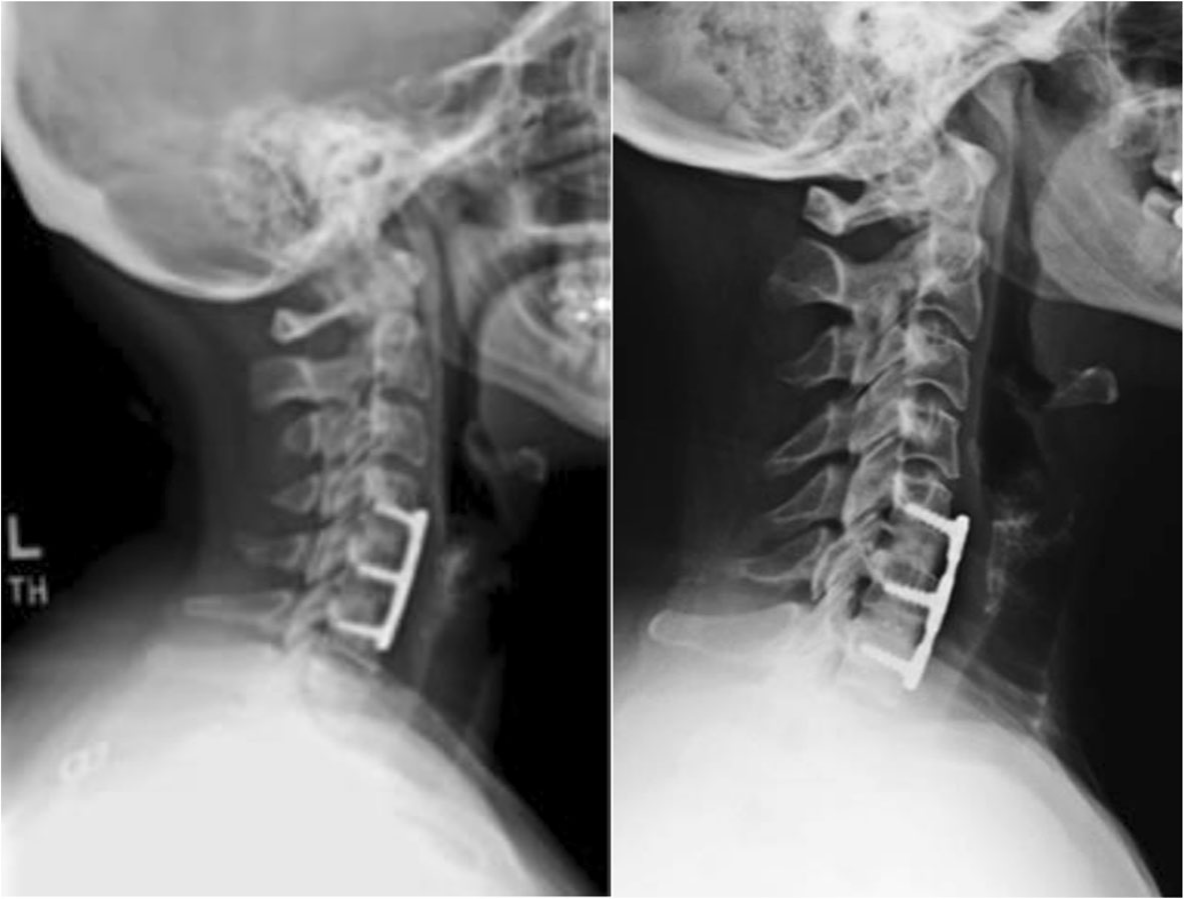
Six months postoperative X-ray images showing fusion for 2-level ACDF treated using frozen allografts (left) and glycerol-preserved (GBP) allografts (right). Reproduced from Rodway and Gander [23] under the Creative Commons Attribution License, which permits unrestricted use, distribution, and reproduction in any medium, provided the original work is properly cited
